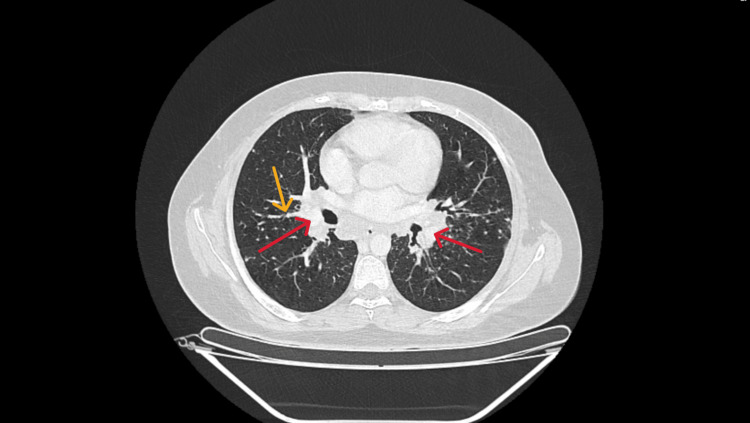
Chest CT scan. Chest CT imaging showing bilateral hilar lymphadenopathy (red arrows) and micronodularity in the lung parenchyma with a perilymphatic distribution (yellow arrow).
